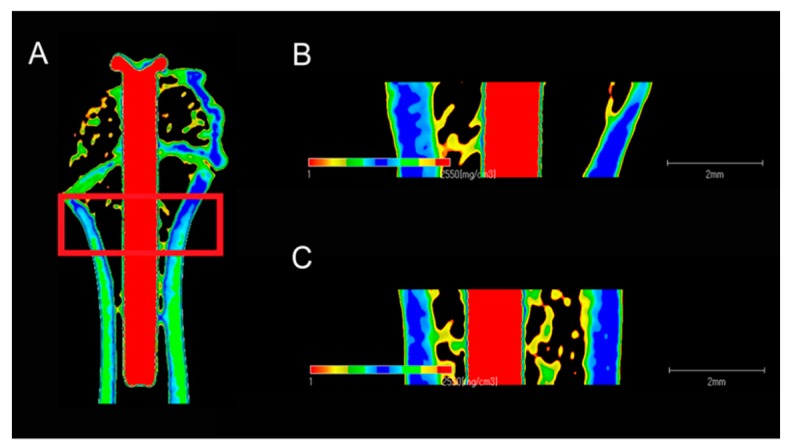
Longitudinal reconstructed microcomputed tomographs (A), of Ti (B) and TNS (C) implants within the region of interest eight weeks after surgery.
